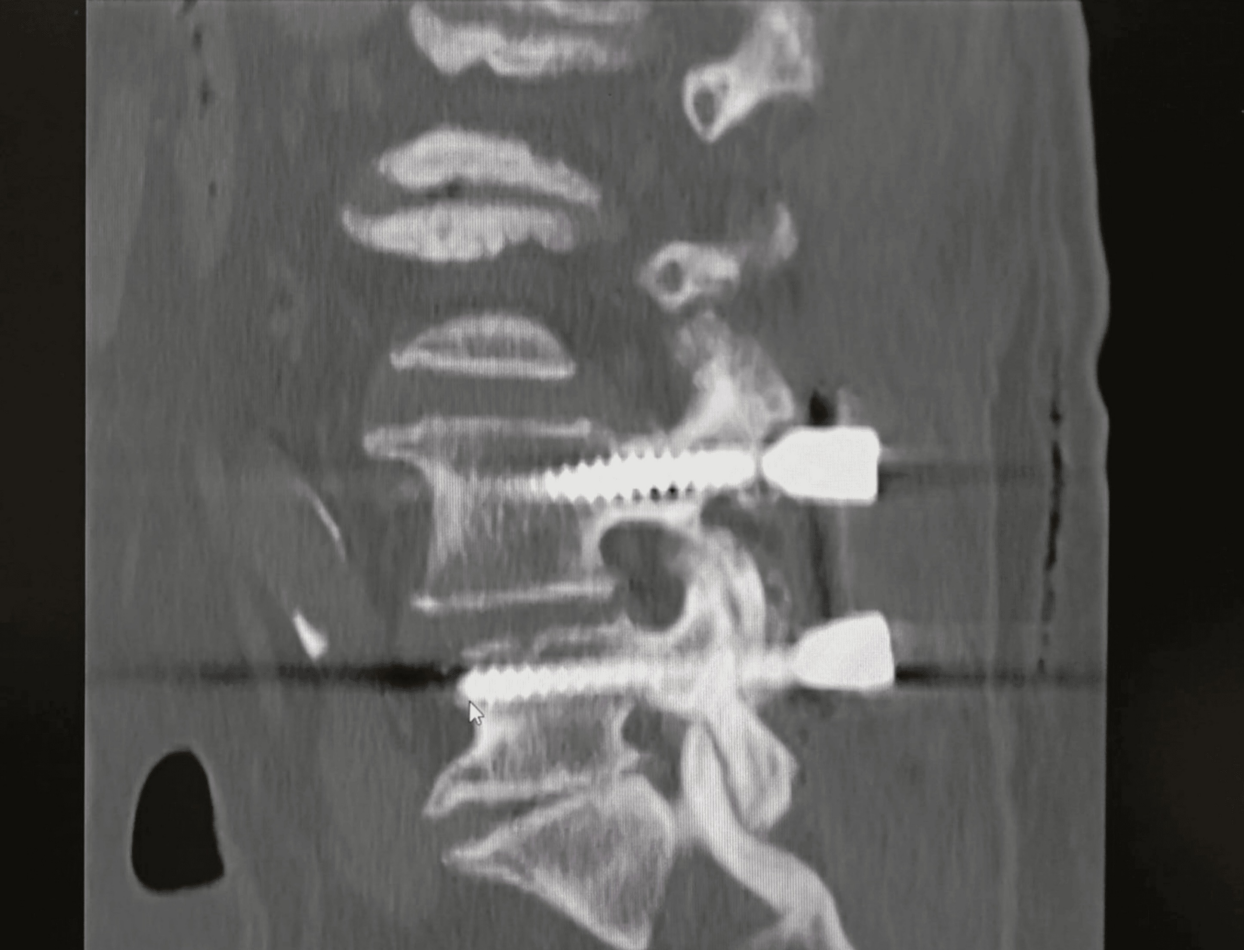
Postoperative spinal CT scan Lumbar sagital CT image showing spinal fusion and instrumentation. Alignment of the spine and placement of the screws seem to be central and symmetrical, which suggests proper positioning.
